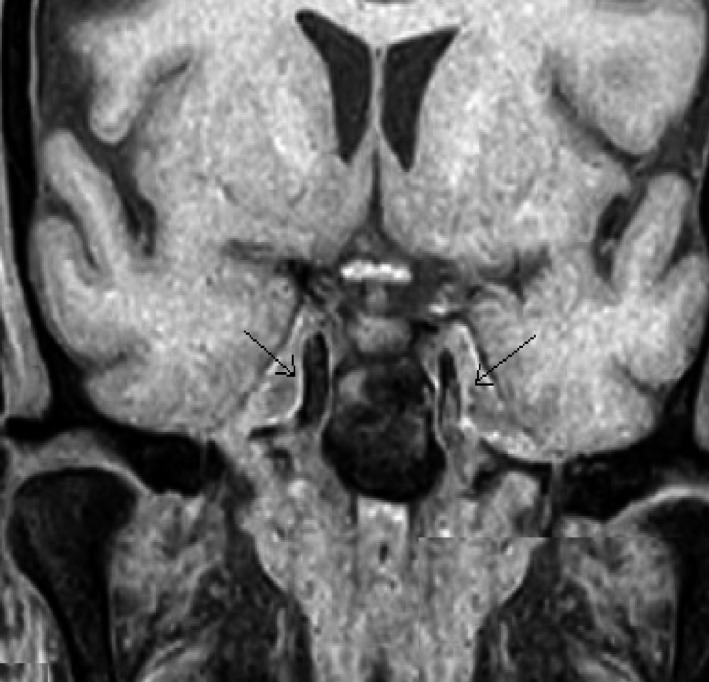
T1 SPIR sequence in MRI with contrast enhancement, showing the presence of internal carotid thrombosis, indicated by the arrow
